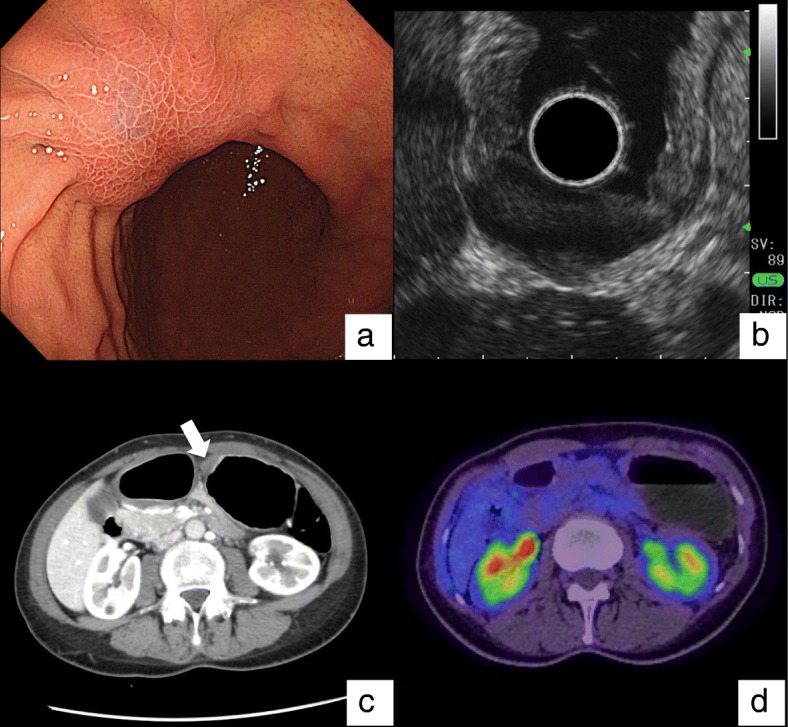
a Endoscopy reveals a flat protrusion in the lesser curvature of lower third of the stomach. b Endoscopic ultrasound shows the tumor thickening the second and third layers of the stomach wall. c Computed tomography (CT) scanning shows the thickened wall at the angle of the stomach (white arrow), but no evidence of tumor metastasis. d Positron emission tomography-CT shows no evidence of tumor metastasis or abnormal uptake in the tumor
